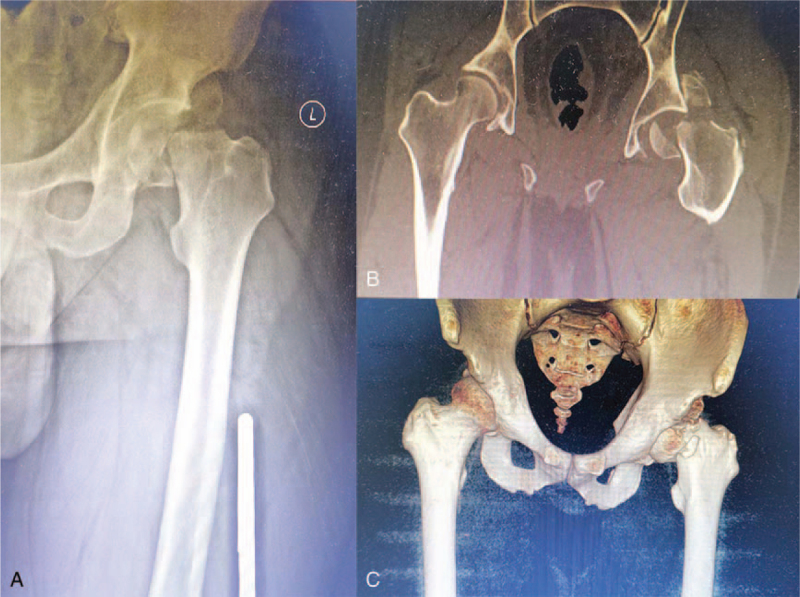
New X-ray and CT examination after closed reduction of hip dislocation. (A) Anteroposterior radiograph of the left hip. (B) Coronal CT images showing femoral head and femoral neck fractures. (C) 3-Dimensional CT images of hip showing femoral head and femoral neck fractures with fragments of the femoral head fracture remained in the acetabular fossa.
